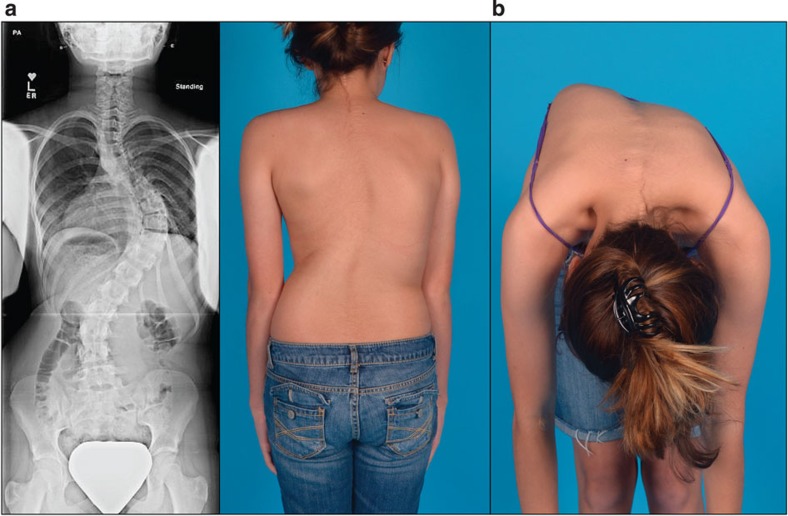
Idiopathic scoliosis in an adolescent female. (a) Lateral radiograph reveals prototypical right thoracic spinal curvature. Shoulder imbalance is evident in clinical photograph. (b) Prominent rib hump is evident on forward bending.
